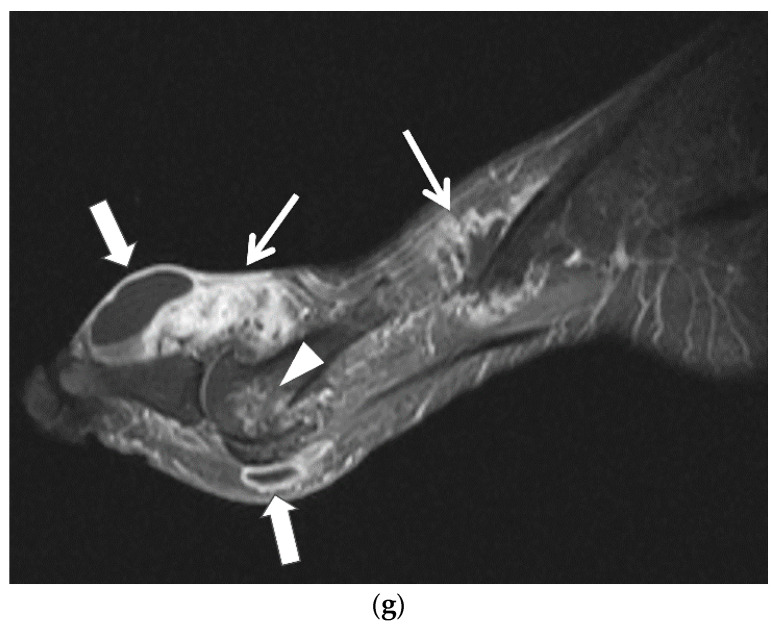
60-year-old man with tophaceous gout of the right foot. (a) AP radiograph of the right foot shows dense nodular soft-tissue thickening at the medial aspect of the first metatarsophalangeal and first tarsometatarsal joints and at the lateral aspect of the fifth tarsometatarsal joint (arrows). (b) Axial and (c) sagittal STIR and (d) axial and (e) sagittal T1-weighted MR images show multiple areas of intermediate-to-low signal intensity in the periarticular regions of the forefoot and midfoot related to MSU deposits and tophaceous gout (white arrows), cortical erosion at the medial aspect of the first metatarsal head (white arrowheads) and cortical erosions between the third and fourth tarsometatarsal joints (dashed white arrows) which show heterogeneous enhancement on the (f) axial and (g) sagittal T1-weighted with fat saturation post-contrast MR images. In (b) note high-signal-intensity lobulated adventitial bursal collection at the lateral aspect of the proximal fifth metatarsal bone (open white arrowheads) which shows intermediate-to-low signal in (d) and rim enhancement in (f). Additional adventitial bursae (white block arrows) are seen at the dorsal and plantar aspect of the first metatarsophalangeal joint, which show high signal in (c), intermediate-to-low signal in (e) and rim enhancement in (g).
